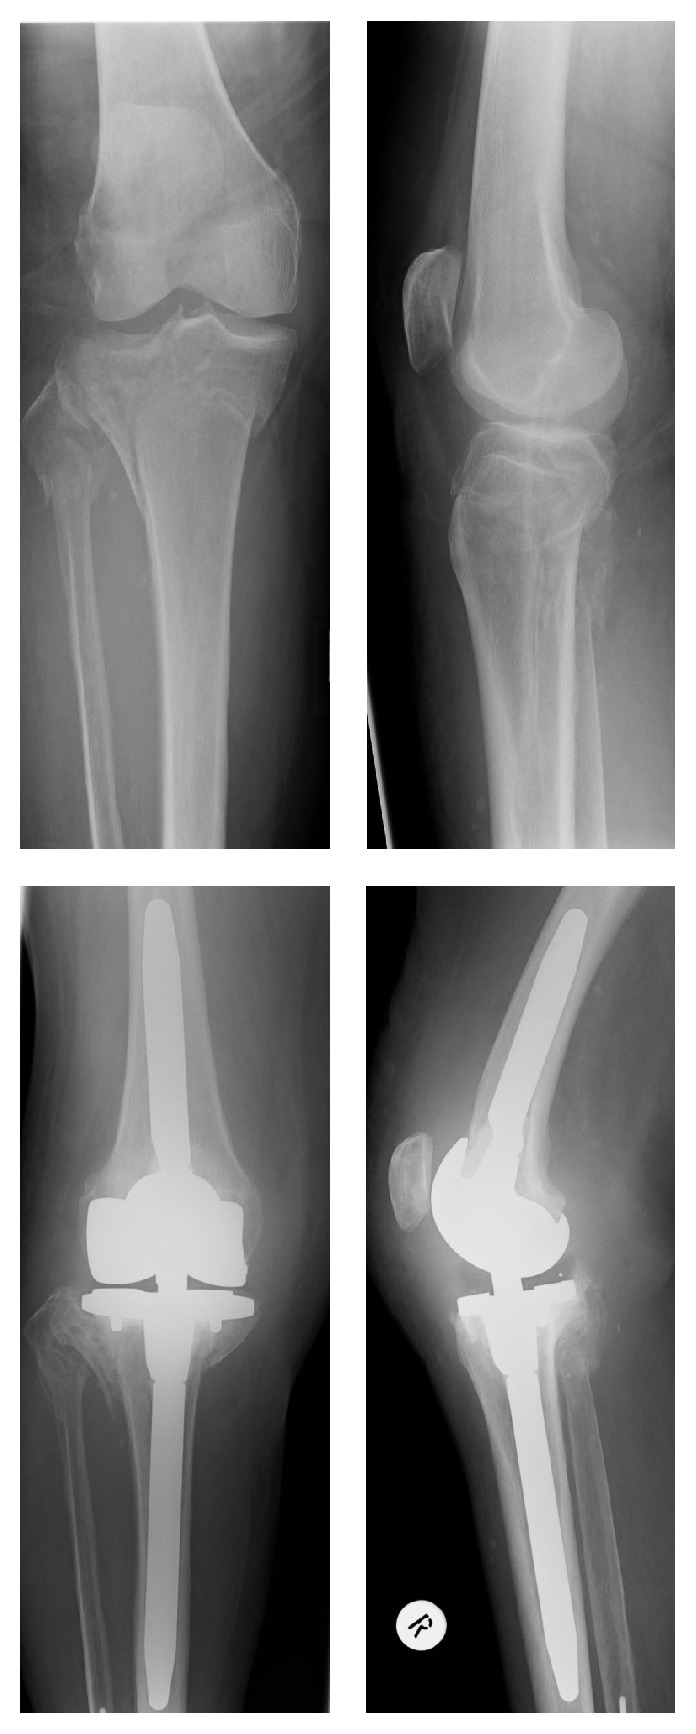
Female, age 86, tibia fracture AO 41C3, Innex SC (Zimmer), postoperative X-ray, KSS1 90, KSS2, and WOMAC 85 (13th month's follow-up).
